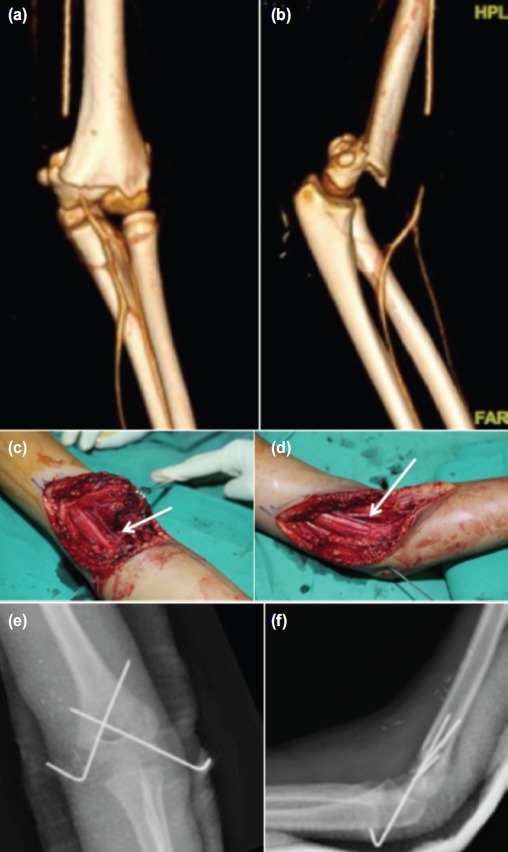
A 12 year-old boy with a supracondylar fracture. Pulses were not palpable and confirmed by Doppler; (a, b) CT angiogram revealed a complete block of the brachial artery with good collateral and distal run-off. (c, d) At operation, the brachial artery was found trapped and kinked by superior bony fragment. It was released and fracture was reduced. (e, f) The fracture was fixed with crossed K wires.
